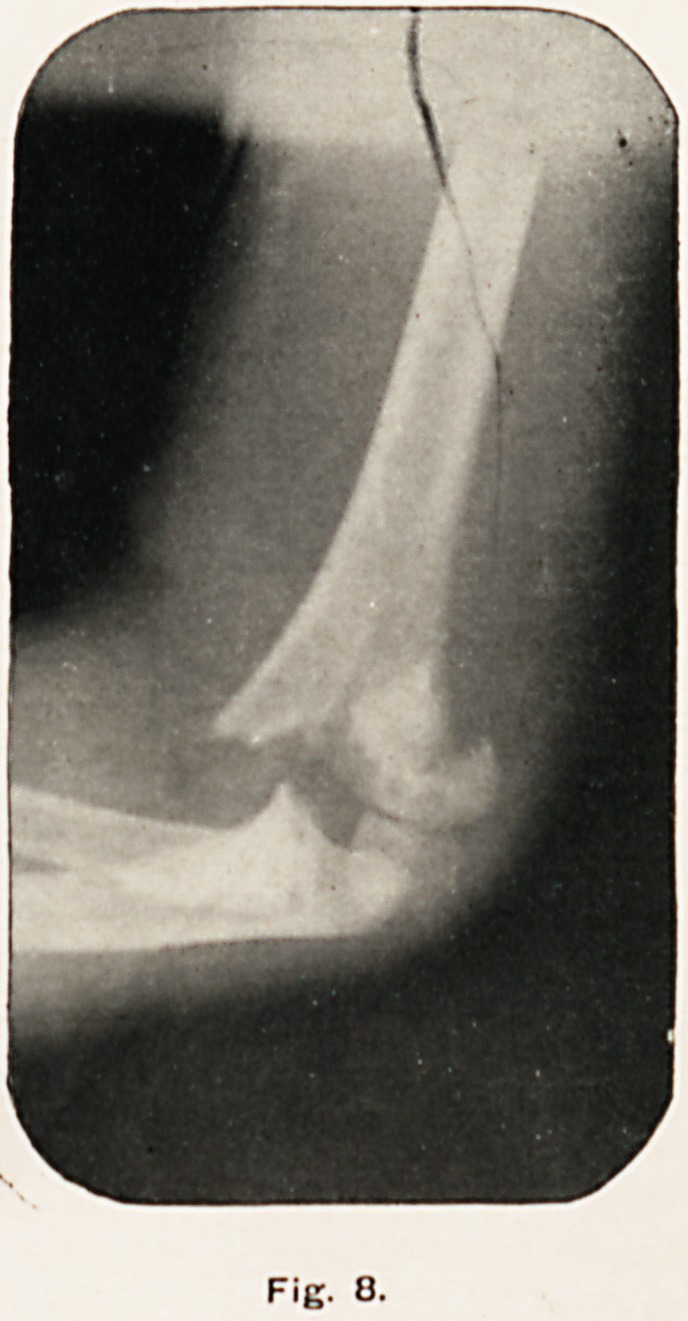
# The X-Ray History of a Fracture
